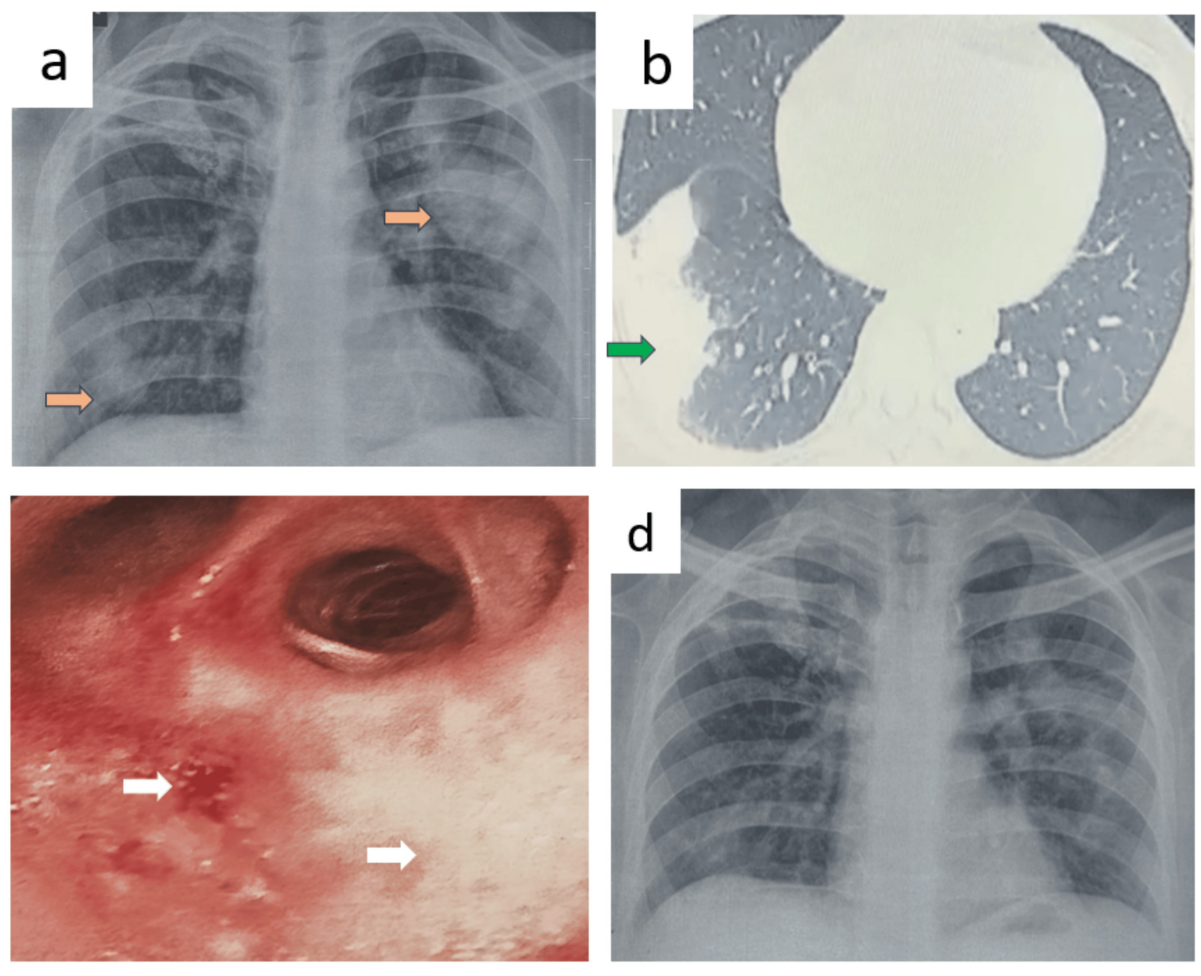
Radiology and bronchoscopy images of patient D before and after treatment. (a) Chest X-ray revealed non-homogenous opacities in the right lower and left upper lung zones marked by brown arrows. (b) CT thorax showed consolidation in the right lower lobe marked by a green arrow. (c) Bronchoscopy showed mucosal ulcerations with whitish membranes and erythema with bleeding spots in the right intermediate bronchus marked by white arrows. (d) After a month, a follow-up chest X-ray demonstrated near-complete resolution of the lung opacities.
